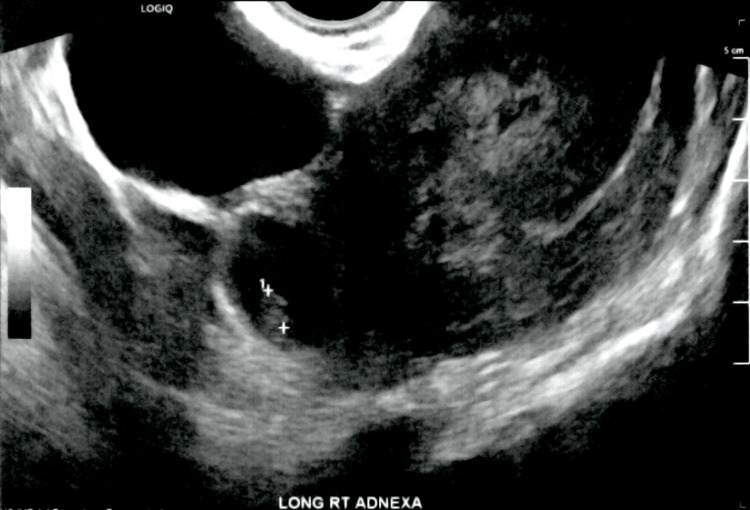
TVUS of the long right adnexa with an ectopic pregnancy measuring 2.3 cm in diameter with crown-rump length measuring 6.9 mm TVUS: transvaginal ultrasound
